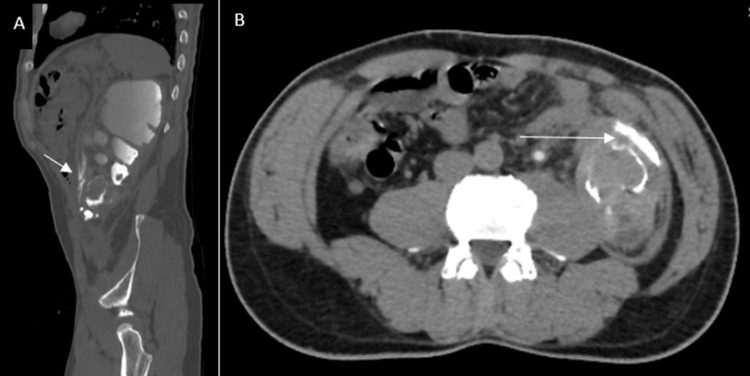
Additional CT images (A) Sagittal CT reformatted image in bone window during the delayed phase showing contrast extravasation tracking around the perirenal hematomas; (B) Axial CT image in the delayed phase, demonstrating contrast medium extravasation adjacent to the lower pole laceration.
